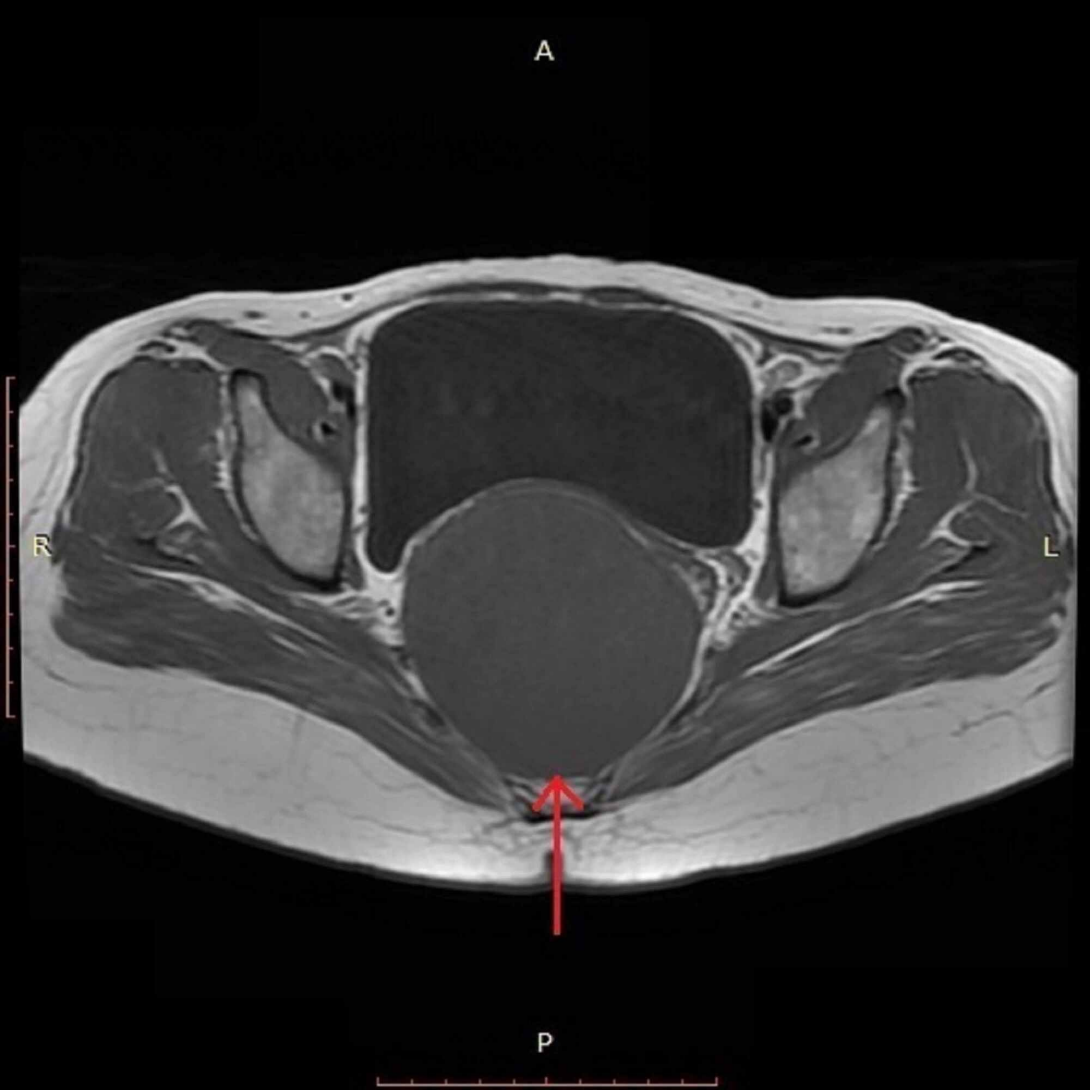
T1W-MRI showing a large, hypointense lesion in the presacral space T1W, T1-weighted; MRI, magnetic resonance imaging
